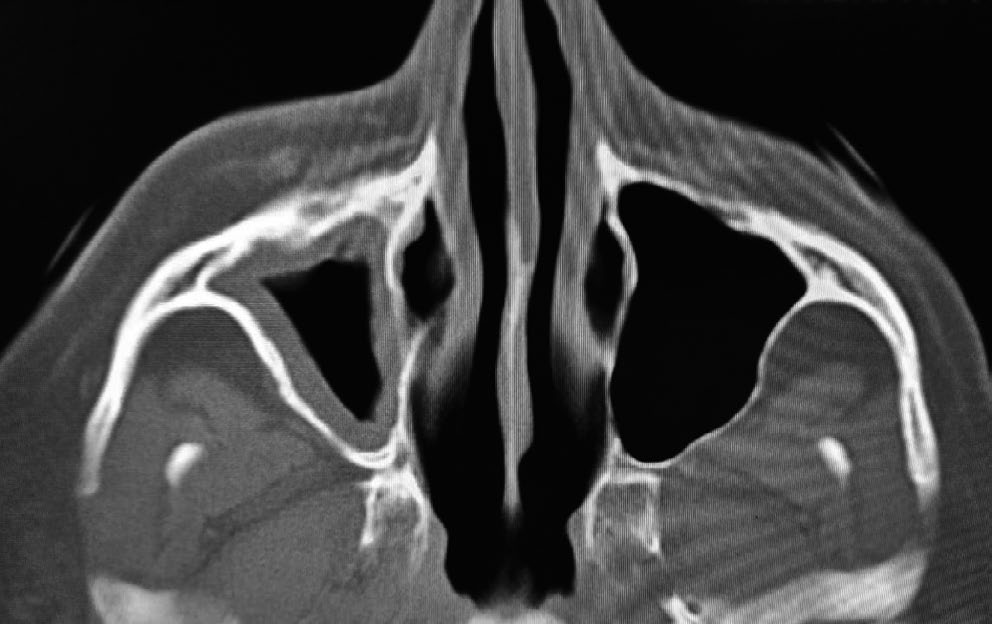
Axial section CT scan reveals maxillary sinusitis
